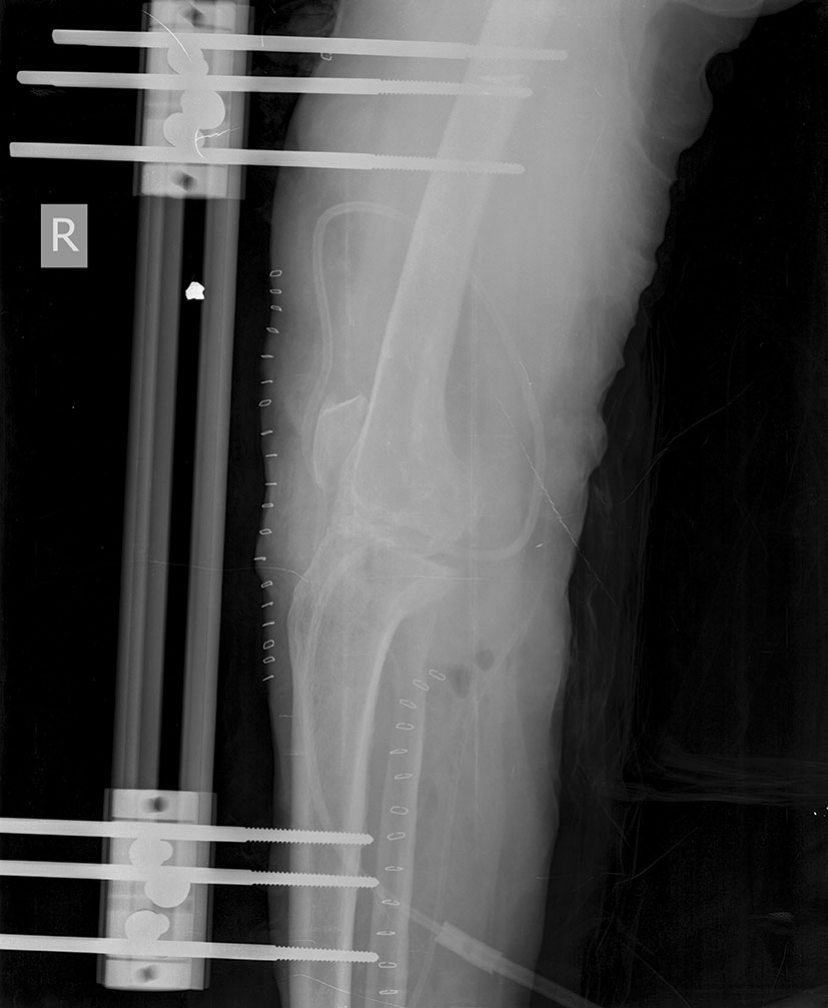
Immediate post-operative lateral plain radiograph showing knee arthrodesis performed with an anterior monorail fixator
